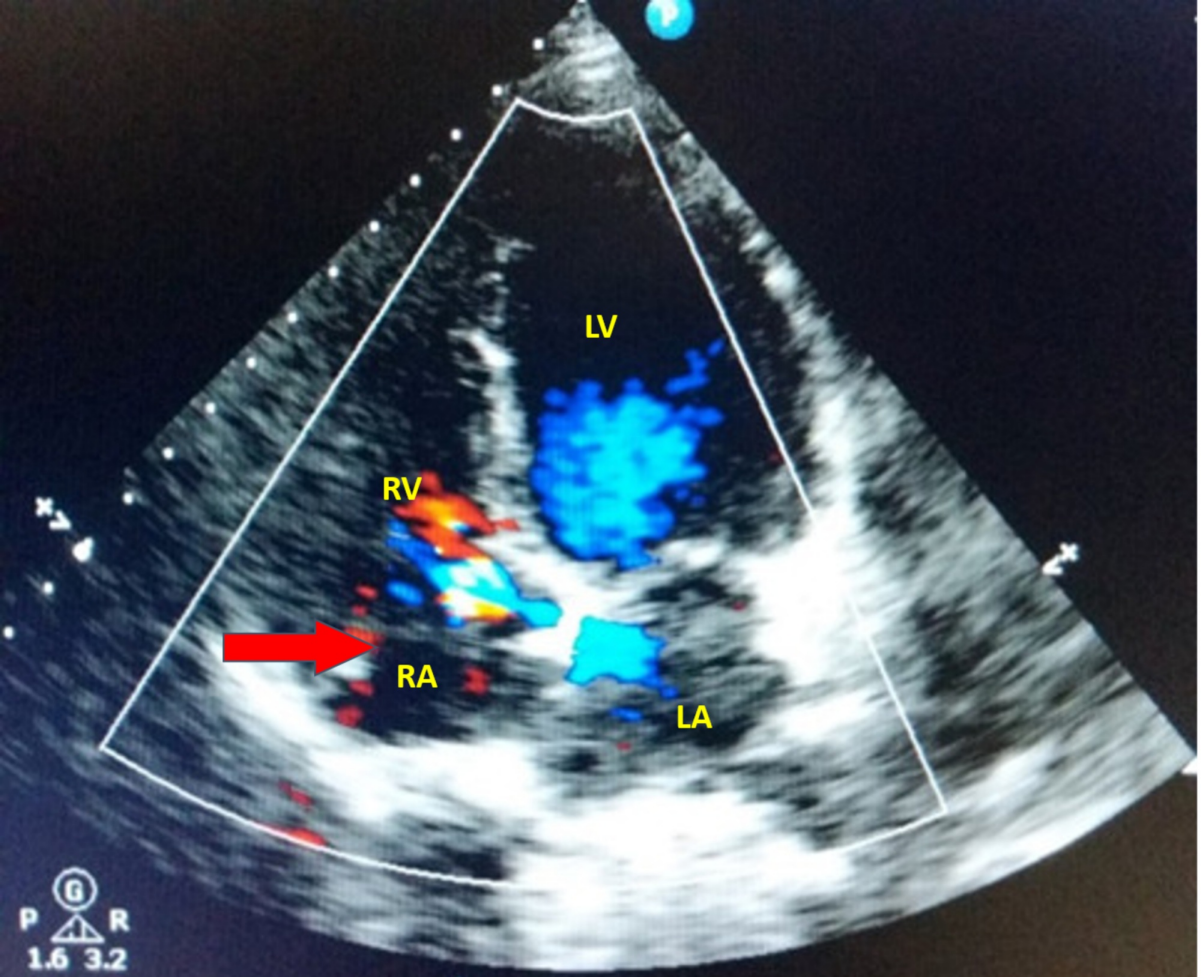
Color Doppler four-chamber view showing a membranous structure (CTD) within the right atrial cavity (red arrow). Abbreviations: LA, left atrium; LV, left ventricle; RA, right atrium; RV, right ventricle
